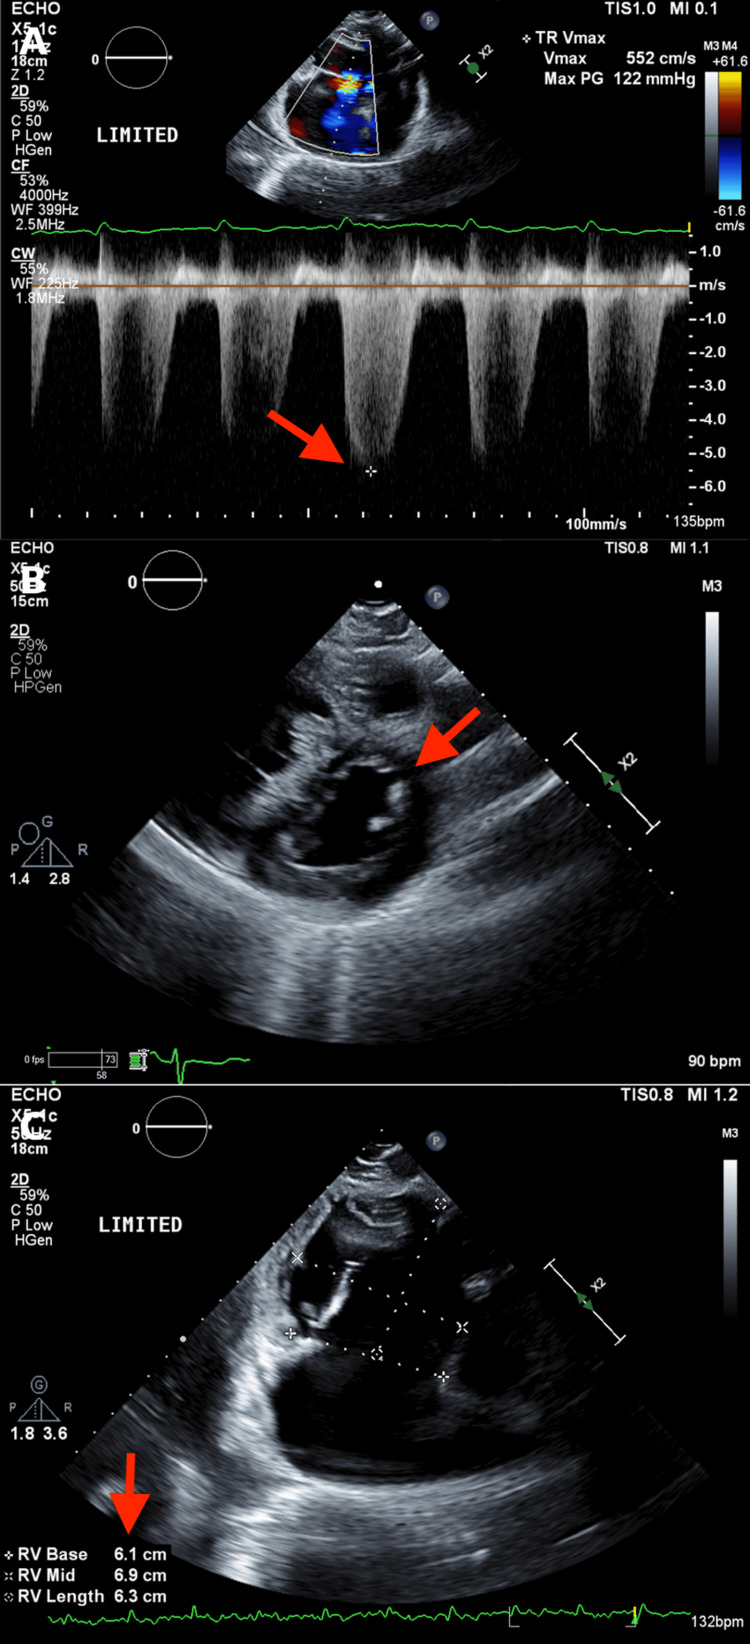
Transthoracic echocardiogram (A) Continuous-wave Doppler across the tricuspid valve showing markedly elevated tricuspid regurgitant velocity (Vmax 5.52 m/s), corresponding to a severely elevated estimated right ventricular systolic pressure. (B) Parasternal short-axis view at the level of the left ventricle demonstrating interventricular septal flattening during systole, consistent with RV pressure overload (D-shaped left ventricle). (C) Apical four-chamber view demonstrating severe right ventricular dilatation with increased RV basal, mid, and longitudinal dimensions. RV: right ventricle; LV: left ventricle; TR: tricuspid regurgitation; Vmax: maximum velocity; PG: pressure gradient
